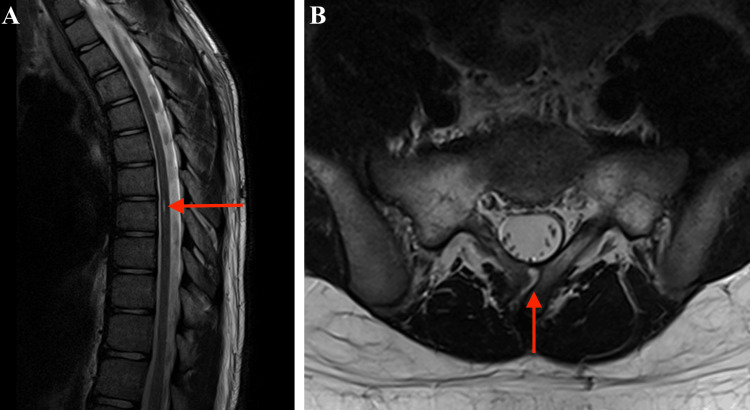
Thoracolumbar spine MRI MRI, magnetic resonance imaging (A) The sagittal plane of the MRI showing a syrinx at the level of the Th8 vertebra (red arrow). (B) The axial plane of the MRI showing a paraspinous cleft of the S1 vertebra on the right side (red arrow).
